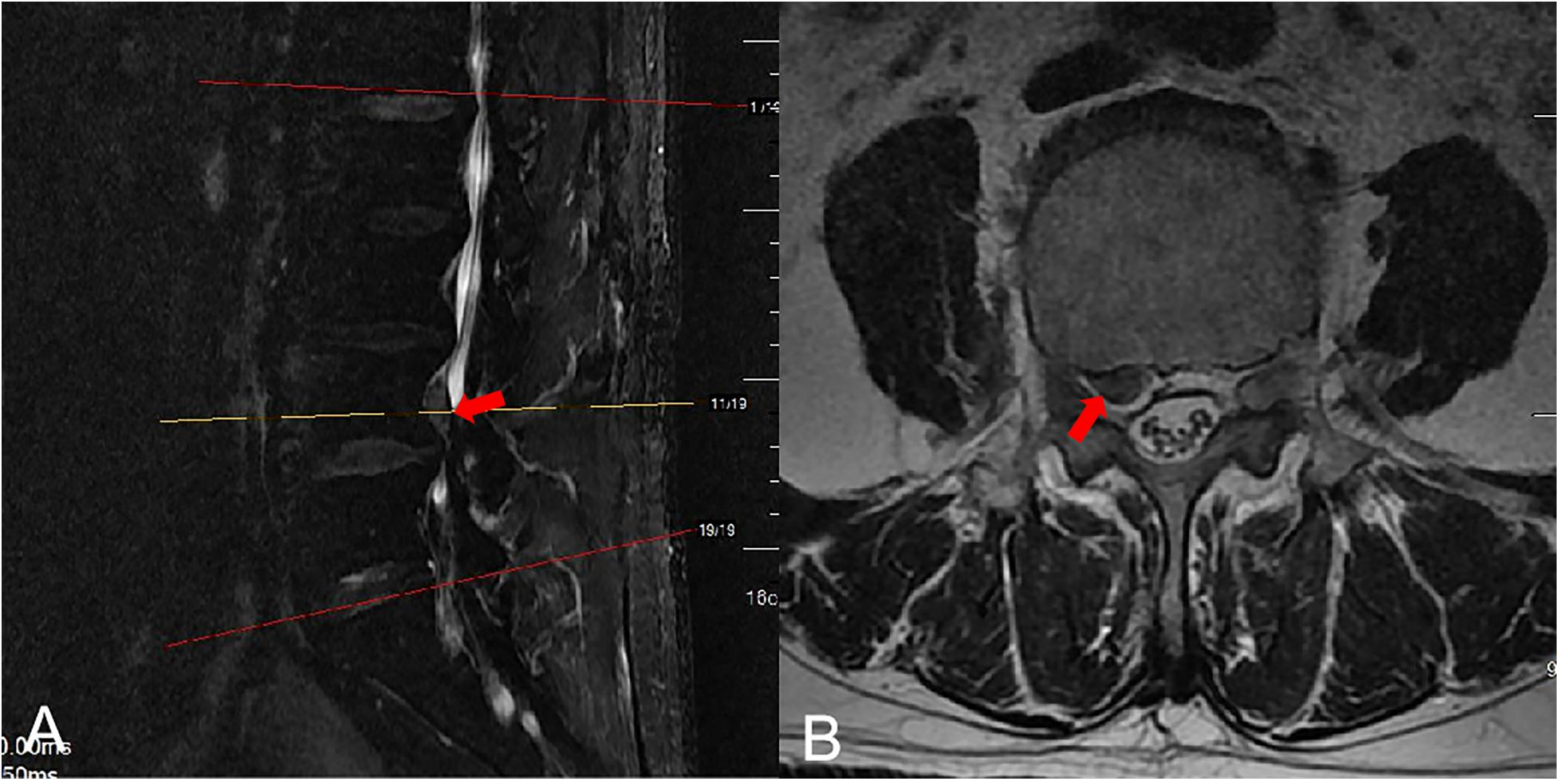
Preoperative MRI of the herniated disc. (A) Sagittal image demonstrated an extruded and sequestered L4/5 disc fragment (red arrow) migrating upward behind L4. (B) Axial image demonstrated the right L4 nerve root is encroached by the herniation (arrow indicates the extruded nucleus pulposus).
